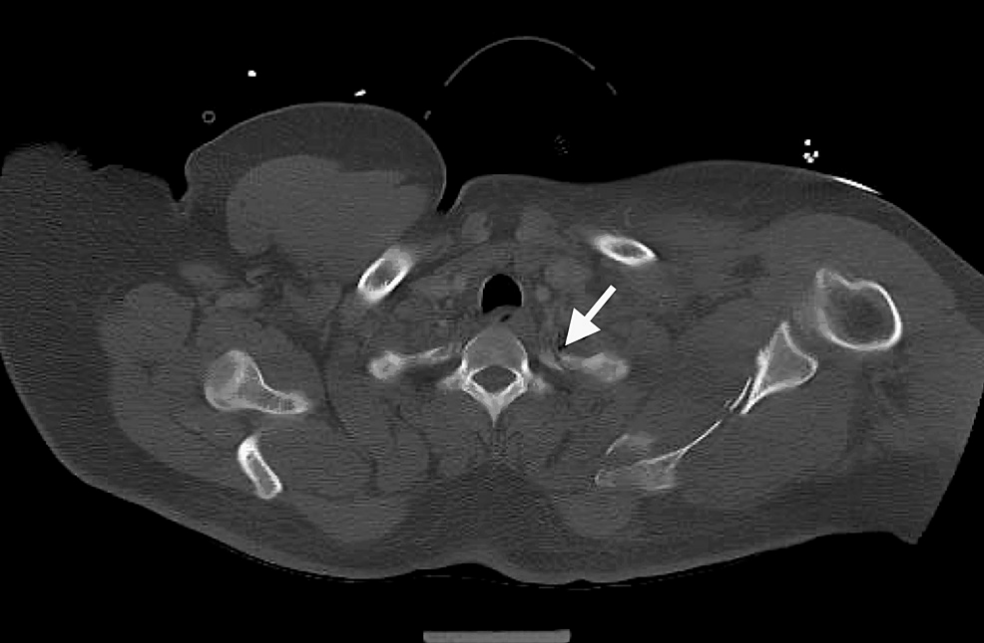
CT scan of the abdomen shows fracture of transverse process of lumbar spine.
